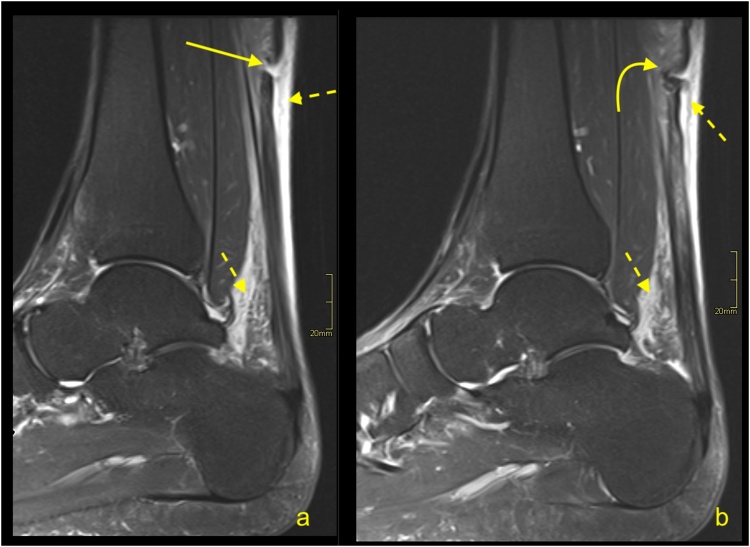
Proximal Achilles tendon rupture. A 32-year-old athlete played squash and felt a sudden pain in the upper part of the Achilles tendon. The Achilles tendon defect is visible (straight arrow) on MRI (a and b); the remaining part of the tendon is wavy-shaped (curved arrow). Reactive changes in the subcutaneous tissue and Kager’s fat pad (dashed arrow). A and b- PD- weighted with fat suppression.
